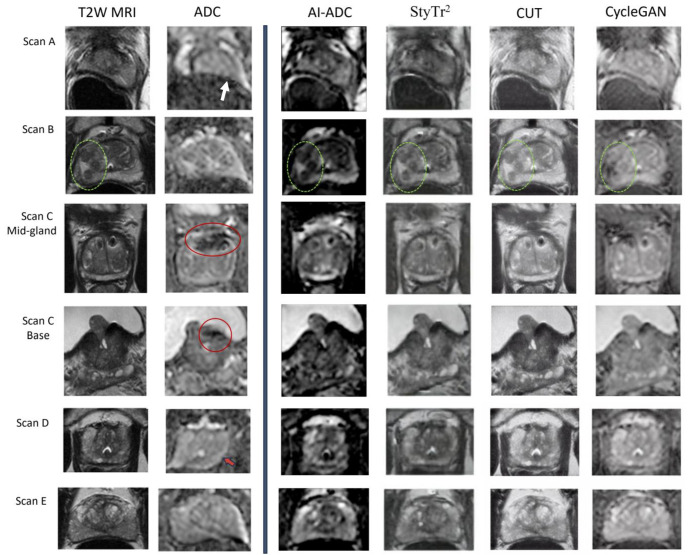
Qualitative MRI results across five different patients. The T2-weighted MRI (T2W MRI) and apparent diffusion coefficient (ADC) maps utilized in clinical evaluations are displayed on the left, and AI-generated ADC maps on the right are provided for comparison. Scan A: The presence of rectal gas significantly compromises the quality of the original ADC map, obscuring the posterior boundary of the prostate (white arrow). The AI-ADC map effectively delineates both the prostate boundary and intraprostatic zones; a comparative level of detail was not achieved by other AI-generated ADC maps. Scan B: The T2W MRI revealed three lesions in the right peripheral zone, which are not visible on the original ADC map. Except for the map produced by CycleGAN, all AI-generated ADC maps demonstrate these lesions (dashed green circles). Scan C: This scan shows a UroLift implant within the mid-to-base anterior transition zone of the prostate. Surrounding this implant, the original ADC map displays a significant artifact (red circle). In contrast, the AI-ADC map reveals detailed intra- and extraprostatic structures with minimal artifact presence. Scan D: In the original ADC map, the left posterior boundary of the prostate gland appears geometrically distorted (red arrow). The AI-generated ADC maps provide an enhanced delineation of prostate zones and outer boundaries. Scan E: The original ADC map shows a poorly defined and stretched left posterior prostate boundary. The AI-generated maps, particularly the AI-ADC, more accurately depict organ boundaries. Notably, the AI-ADC map more clearly highlights the hypointense capsule of a benign prostatic hyperplasia nodule in the left transition zone, compared to other generated images.
